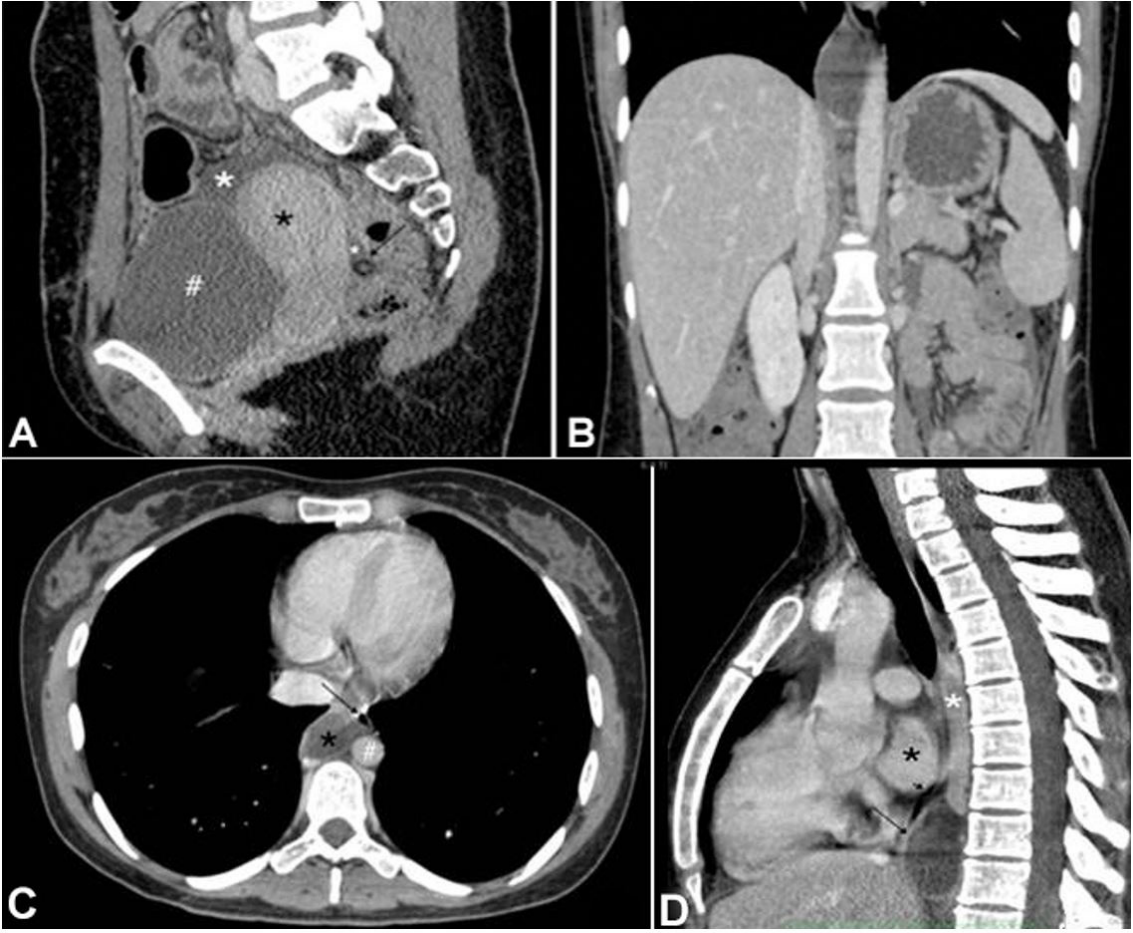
Contrast-enhanced CT scan of the abdomen after drainage, A – shows a significant reduction in ascites (white asterisk) and partially drainage (arrow) in the sagittal plane, in addition to the uterus (black asterisk) and the bladder (#); B – the coronal section image, confirms the total regression of ascites detected in the admission exam; C and D – Contrast-enhanced chest CT scan after drainage, C - axial section, and mediastinal window shows the close relationship between the cyst and the thoracic duct (black asterisk) and other structures such as the descending aorta (#), the azygous vein (white asterisk) and the esophagus (arrowhead); it also reveals the absence of pleural effusion; D - sagittal section shows the major diameter of the cyst at the site of the thoracic duct (long arrow), its location in the posterior mediastinum and the relationship with the esophagus (short arrow), the right ventricle (black asterisk), and the azygous vein (white asterisk).
